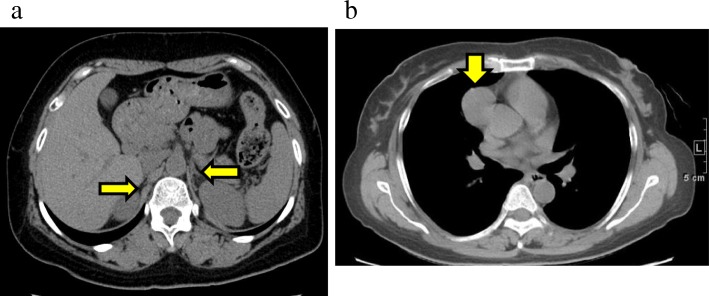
a On an abdominal CT, atrophy of the bilateral adrenal glands was seen (arrows). b A solid mass was seen in the anterior mediastinum (arrow) on a chest CT, suspected to be thymoma (34 × 34 mm in size)
